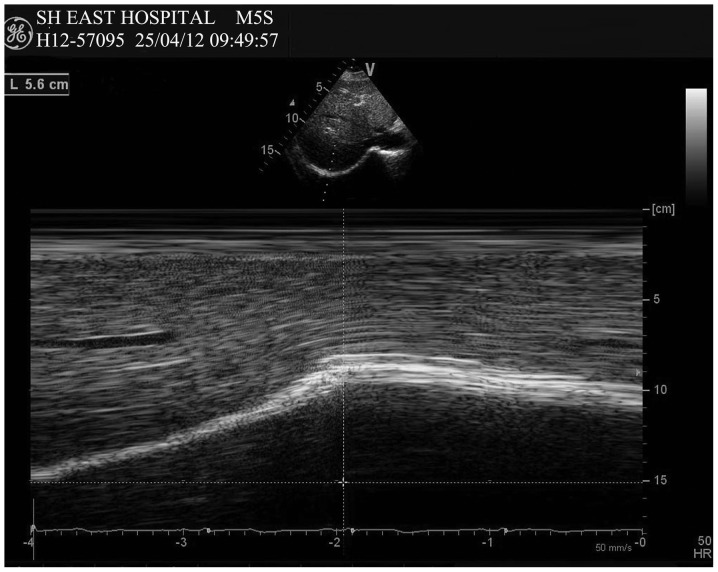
M-mode US of forced breathing and corresponding cardiac cycle under the hyper-echogenic curve in a healthy subject. US, ultrasound.
